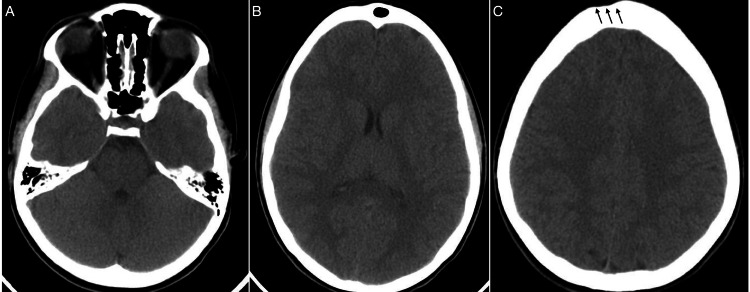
CT scan with no contrast after the treatment of Pott’s puffy tumor. CT scan after one year of treatment showed the absence of sinusitis with regular pneumatization of the frontal and ethmoidal sinuses (A). Expansion of the frontal lobe near the inner frontal protuberance (B) and a tiny depression on the surface of the frontal bone (triple black arrows) (C).
